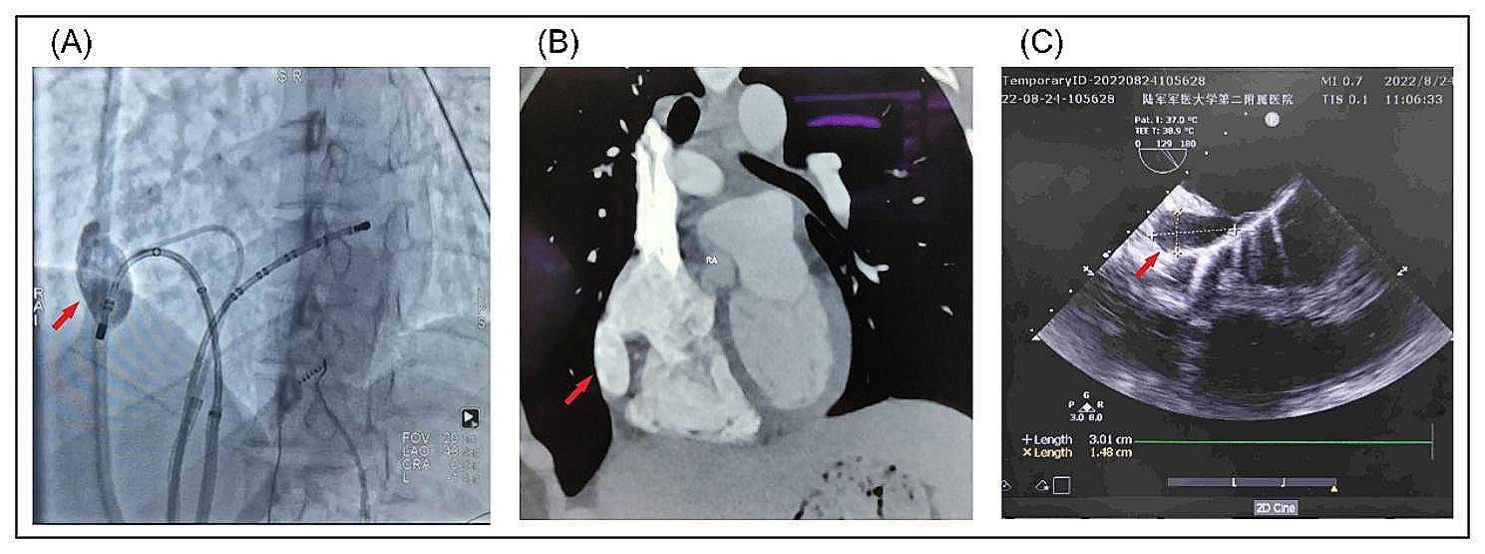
(A) Selective angiography showing a smooth sac-like abnormal structure in the right atrial free wall with no commissural muscle showing (red arrow). (B&C) CTA and TEE showing a diverticulum originating from the right atrial appendage (red arrow). CTA: computed tomography angiography; TEE: Transesophageal echocardiography
